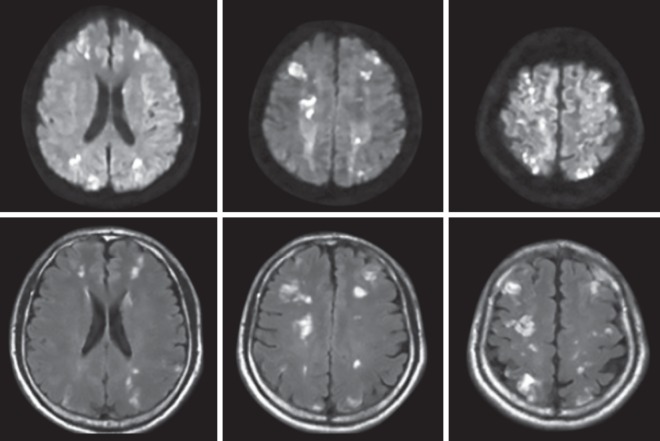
MRI brain of case 1 showing multiple infarcts
